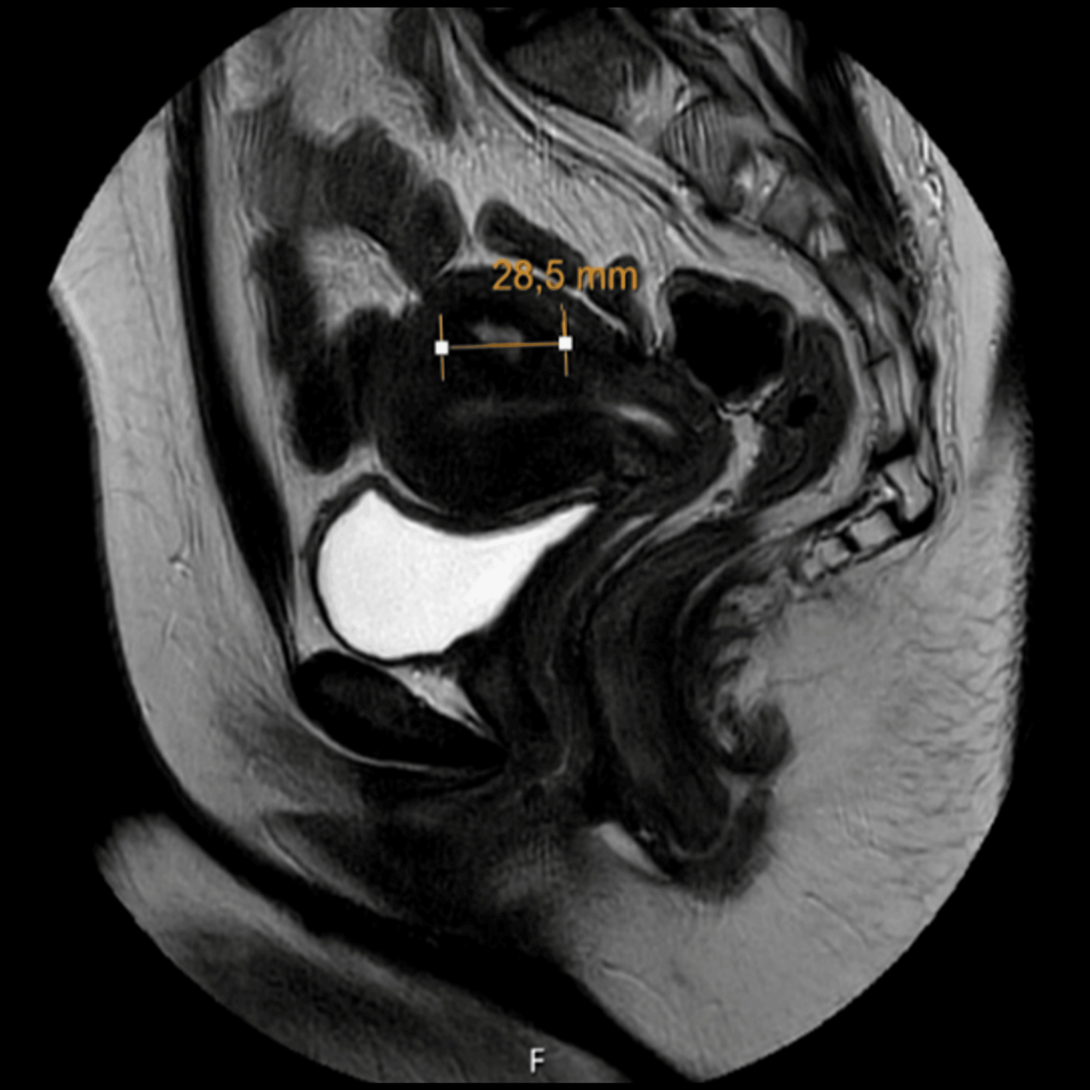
MRI at three months follow-up
